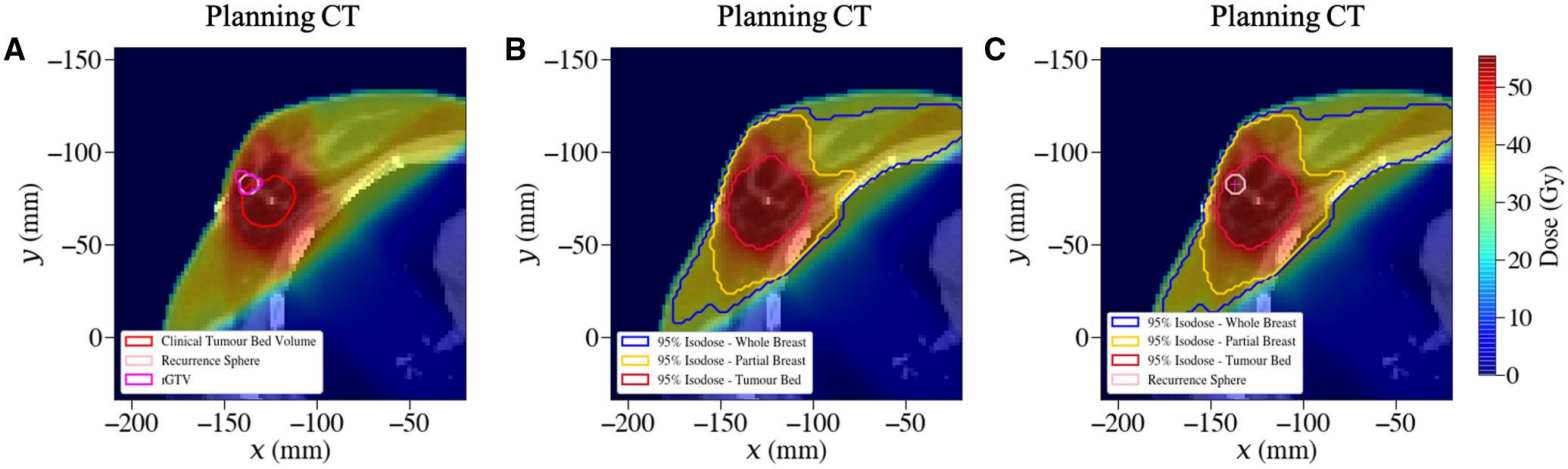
Recurrence structure sets and dose structures for Patient X. (A) Clinical tumour bed volume, mapped rGTV volume as outlined by the radiation oncologist, and the rGTV sphere generated around the mapped rGTV centroid. (B) 95% dose structures generated from the planned treatment dose distribution for 95% dose to high-risk (TB), intermediate-risk (PB) and low-risk (WB) regions to capture dose spread outside clinical CTVs. (C) The planning CT with overlaid 95% dose structures and spatially mapped recurrence sphere and centroid. This demonstrates the difference in classification between a CTV-based comparison (A) and dose structure comparison (C).
